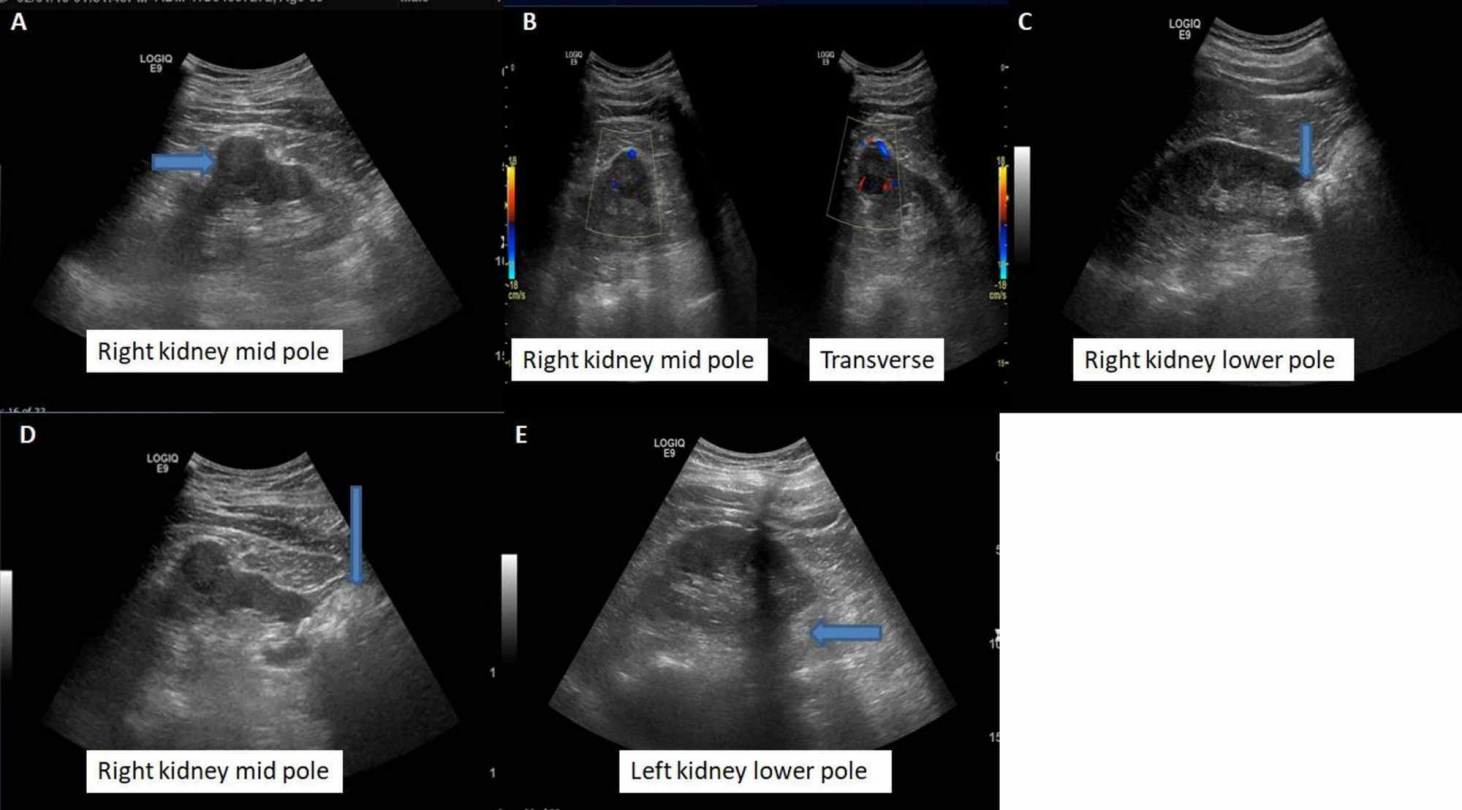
Ultrasound of the abdomen The right kidney demonstrates a hypoechoic mass lesion in its middle pole showing internal and peripheral vascularity measuring 25 x 22 mm (A and B). An ill-defined focal mass lesion can be seen in the right kidney lower pole with calcifications and posterior acoustic shadowing (C and D). A subtle hypoechoic round-shaped lesion can be seen at the medial aspect of the left kidney (E).
